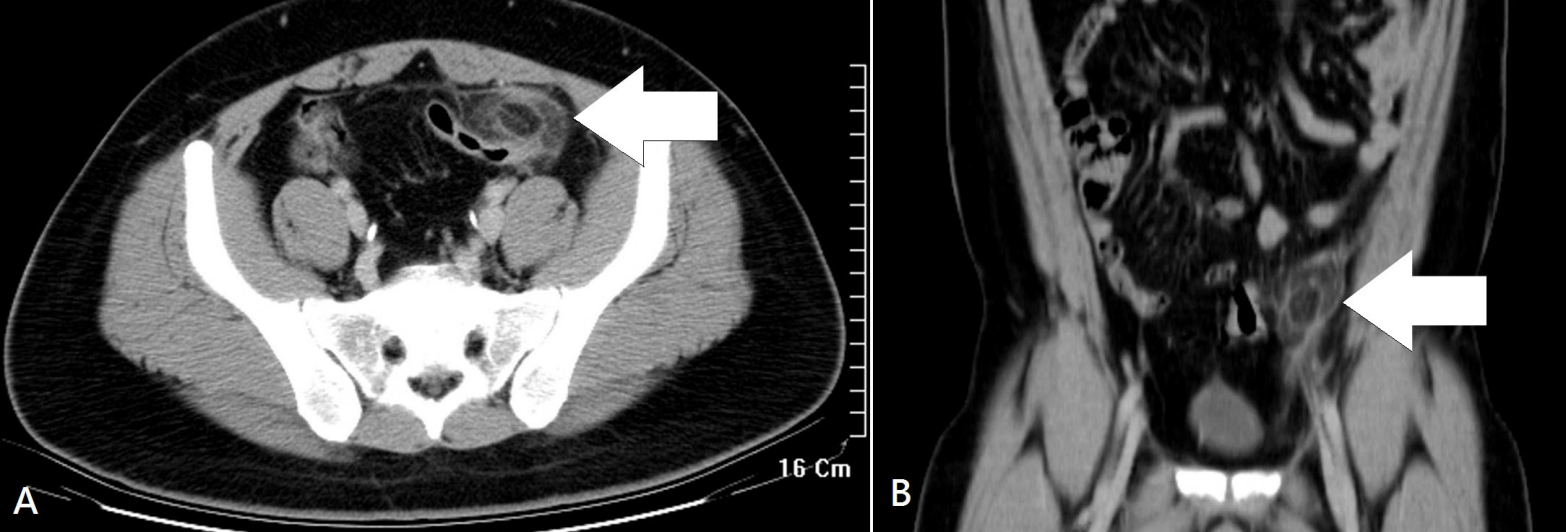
A) Axial and B) coronal abdominal computed tomography showed a pericolonic oval mass (arrow) with a hyperattenuating rim and surrounding fat stranding. A central hyperdense linear lesion corresponded to the thrombosed draining vein.
